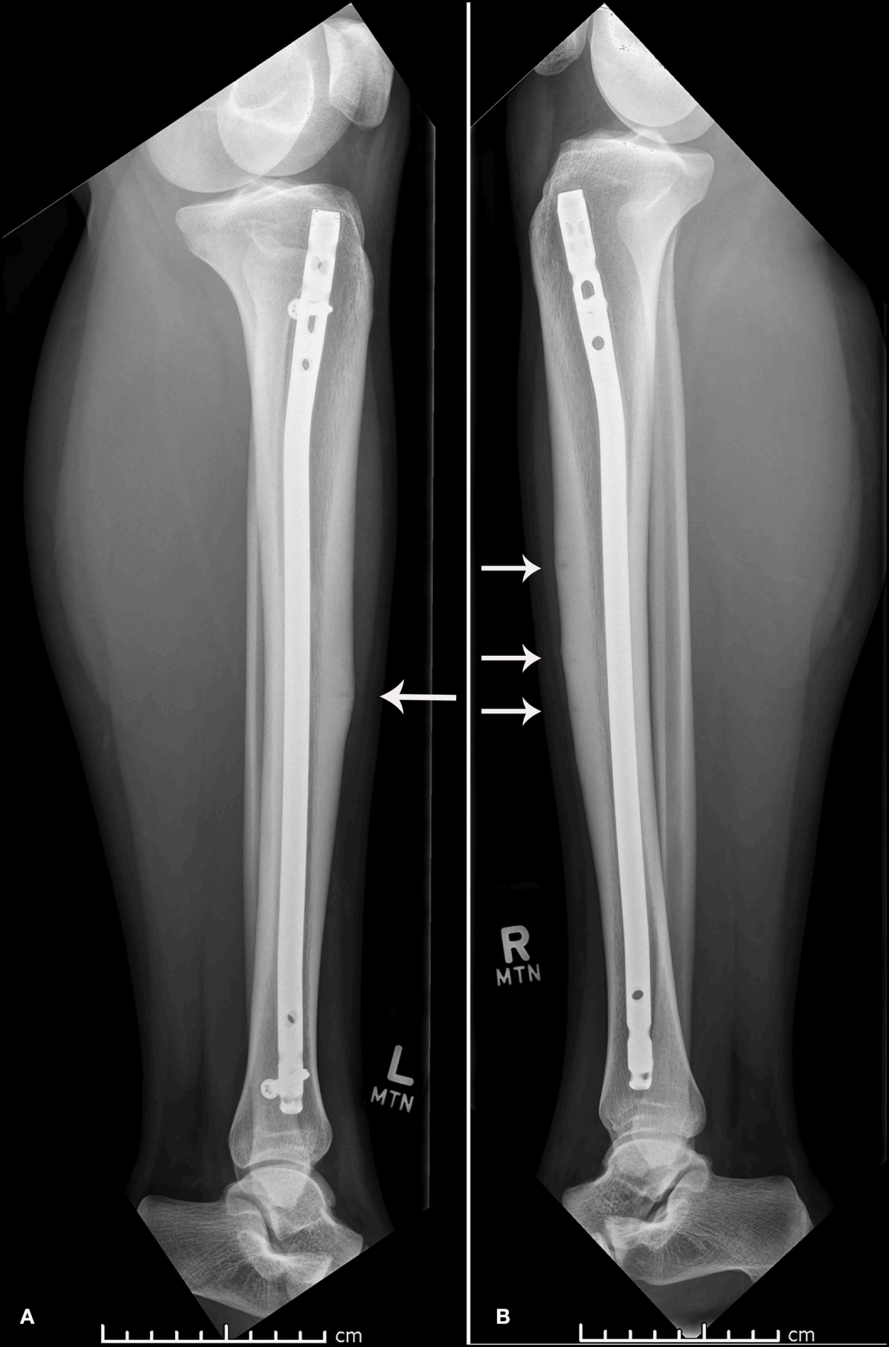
One year post-operative lateral x-ray of the left (A) and right (B) tibia and fibula.
